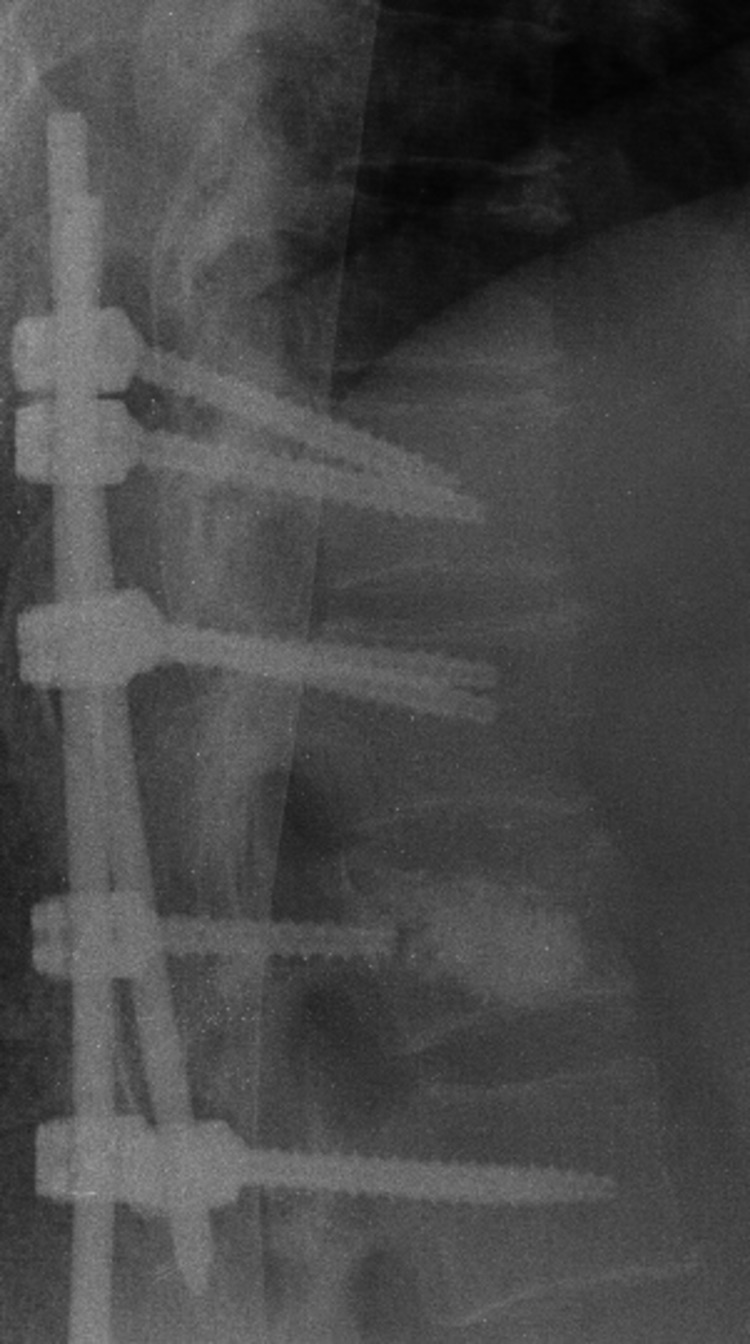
Lateral roentgenogram after balloon kyphoplasty and spinal instrumentation.
